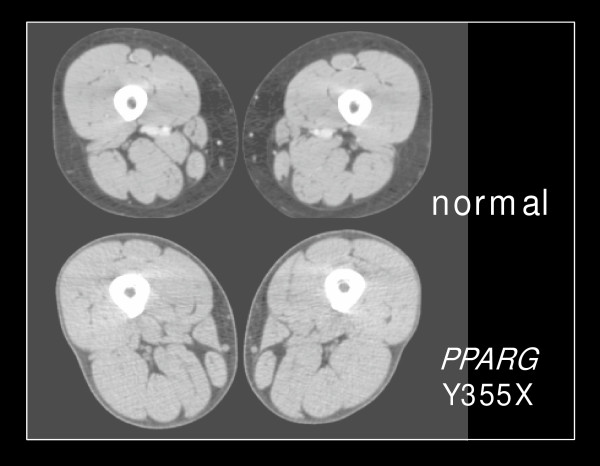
Selected imaging studies from the FPLD3 subject. Panel shows computed tomography cross-section of upper thigh of a normal individual and subject II-5, who had markedly decreased subcutaneous fat on both extremities.
